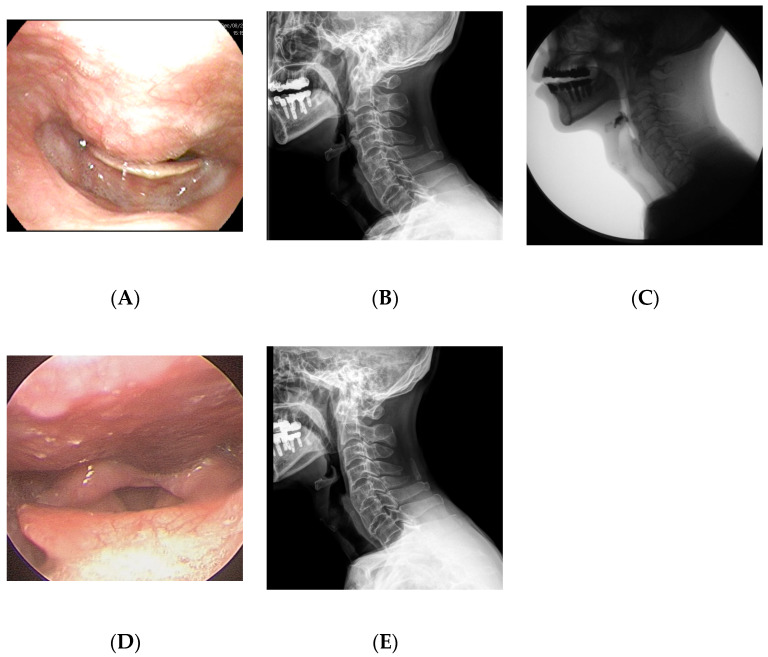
A 65-year-old man presented with dysphagia and odynophagia for the past 3 months and 12 kg weight loss. (A) Preoperative laryngoscopy revealed a protruding mass at the posterior pharyngeal wall obstructing the laryngeal entrance. (B) Lateral cervical radiograph showed a bridging osteophyte at the anterior cervical and thoracic vertebral bodies, suggesting DISH. (C) Preoperative visual fluoroscopic swallowing study revealed moderate dysphagia in the oral and pharyngeal phases with incomplete closure of the epiglottis. (D) Postoperative laryngoscopy showed disappearance of the protruding mass at the posterior pharyngeal wall. (E) There was no evidence of regrowth of the osteophyte at 1-year postoperative radiograph.
